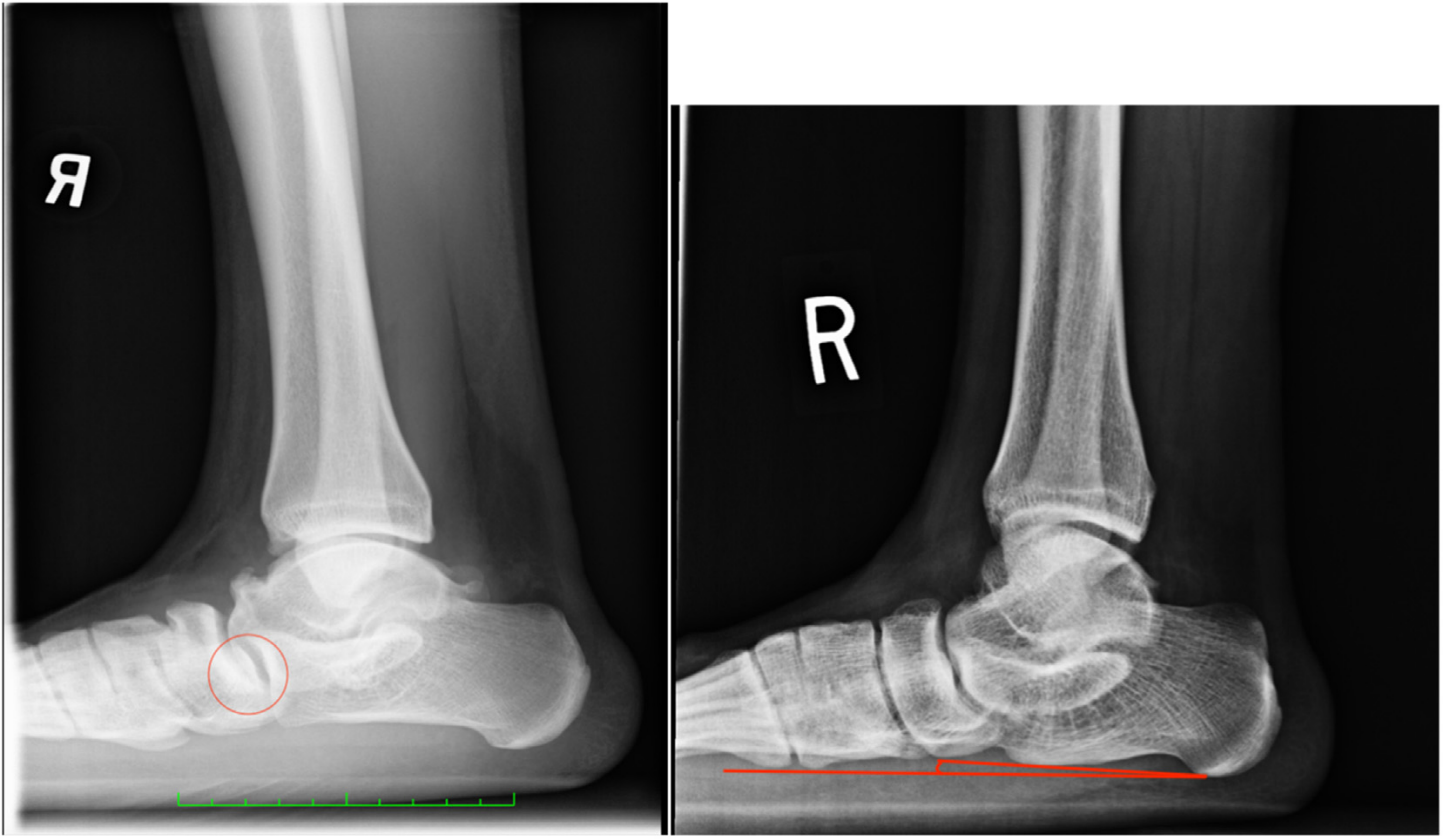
Talonavicular sclerosis/changes (left) and pes planus (left and right). Both are right ankle, lateral radiographs.
